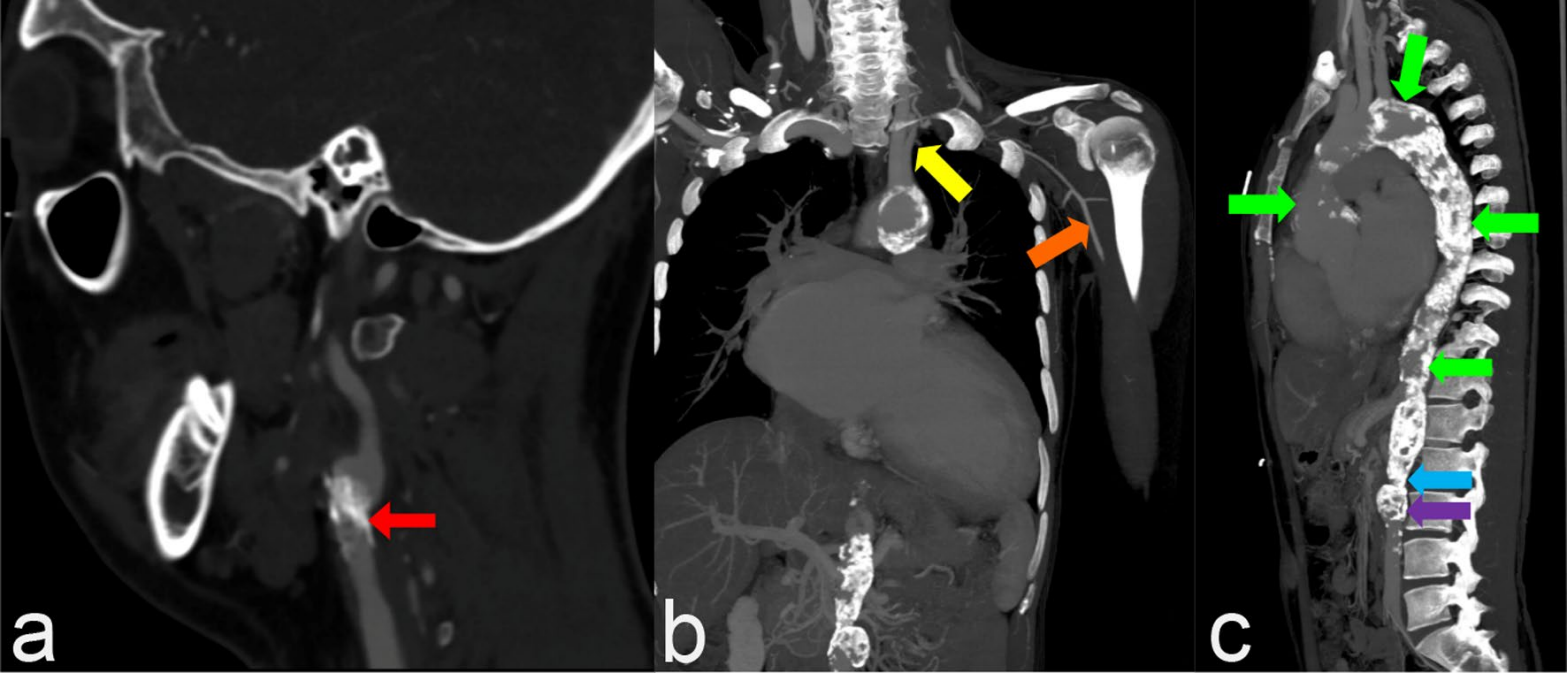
CTA examination showed a calcification in the left common carotid artery (red arrow) leading to stenosis; b total occlusion of the left subclavian artery (yellow arrow) with the left axillary artery (orange arrow) receiving vascularization from the collateral artery of the common carotid artery; (c) calcification of the peri-aortic valve, ascending aorta, aortic arch, descending aorta, and abdominal aorta (green arrow) with high-grade stenosis on the inferior side of the renal artery branching (blue arrow) accompanied by a post-stenotic dilatation (purple arrow)
